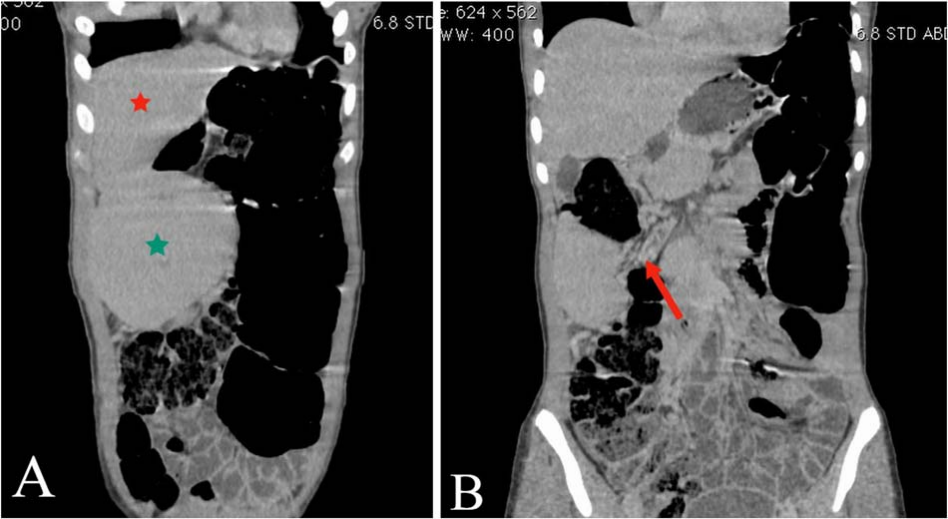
(A and B) The coronal plane of the abdominal CT reveals the liver (A, red star) and the WS in the right upper quadrant (A, green star), with torsion evident in the splenic mesentery (B, arrow).
